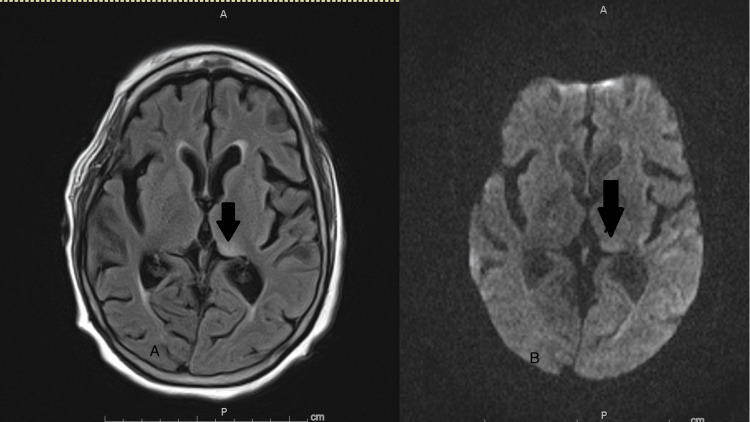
Progressive findings on MRI brain MRI brain showing area of progressing hyper-intensity affecting the posterior left thalamus in fluid attenuation inversion recovery (FLAIR) sequence (arrow in A) with a corresponding diffusion restriction (arrow in B).
